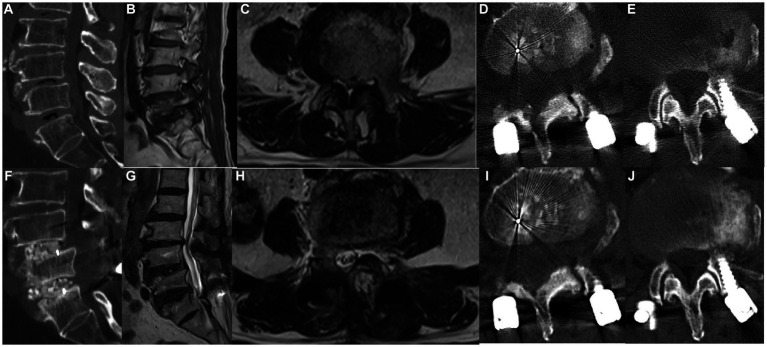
A 58-year-old woman underwent MHSPS TLIF for L4/5 lumbar spinal stenosis with instability and unilateral lower extremity pain. Preoperative sagittal computed tomography confirmed segmental instability (A). Preoperative sagittal (B) and axial (C) T2-weighted magnetic resonance imaging shows lumbar spinal stenosis and instability. Three days after surgery, axial computed tomography shows the split spinous process of two levels (D,E). Postoperative sagittal computed tomography 1 year after surgery shows a robust interbody fusion (F). Axial (G) and sagittal (H) T2-weighted imaging 1 year after surgery shows good decompression and no marked difference in the cross-sectional area of the paraspinal muscles. Axial computed tomography 1 year after surgery shows a bony union of the spinous process of two levels (I,J).
